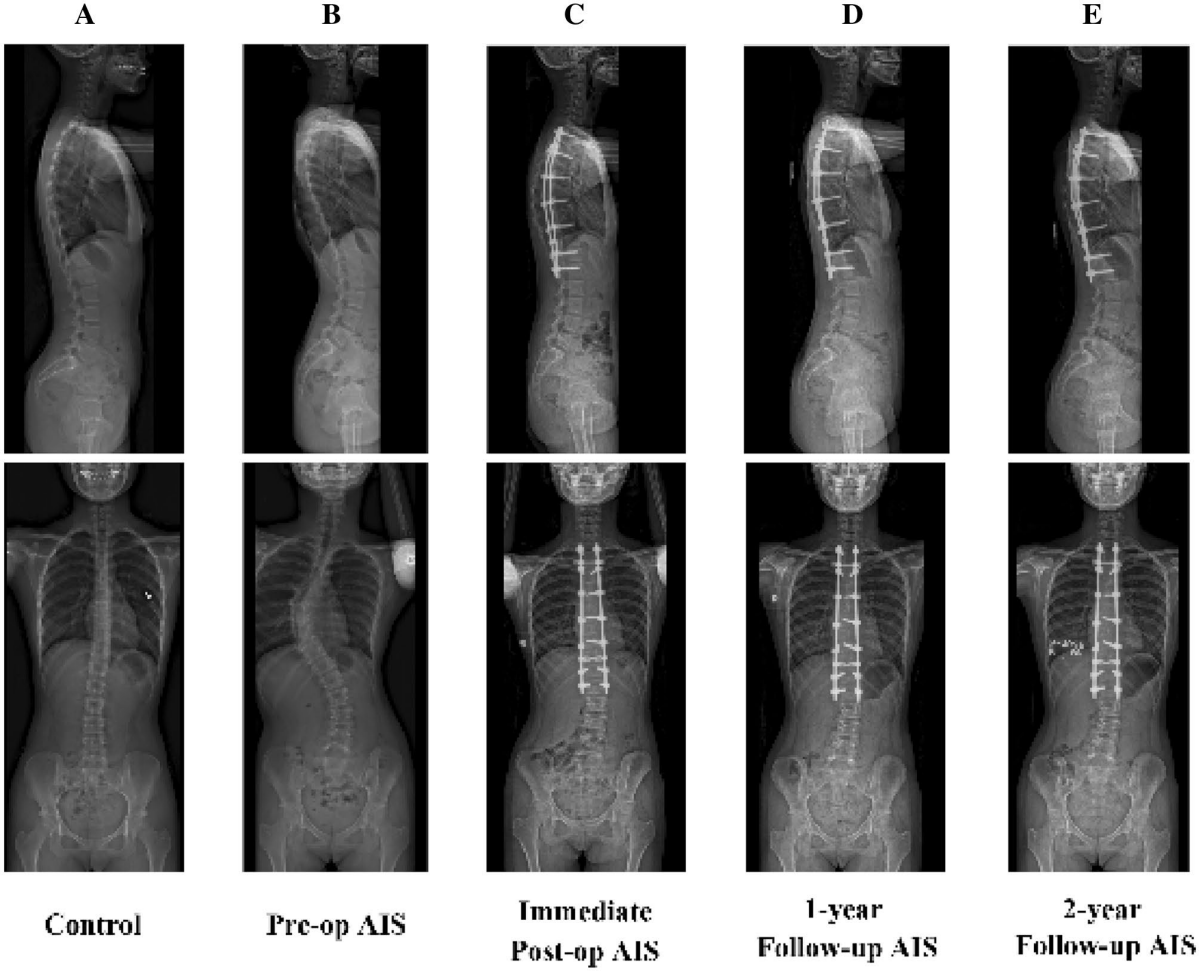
Sagittal radiographs of an asymptomatic female control (A) and a female patient with adolescent idiopathic scoliosis taken pre-operatively (B), and immediate post-operatively (C), at 1-year (D) and 2-year (E) follow-up.
